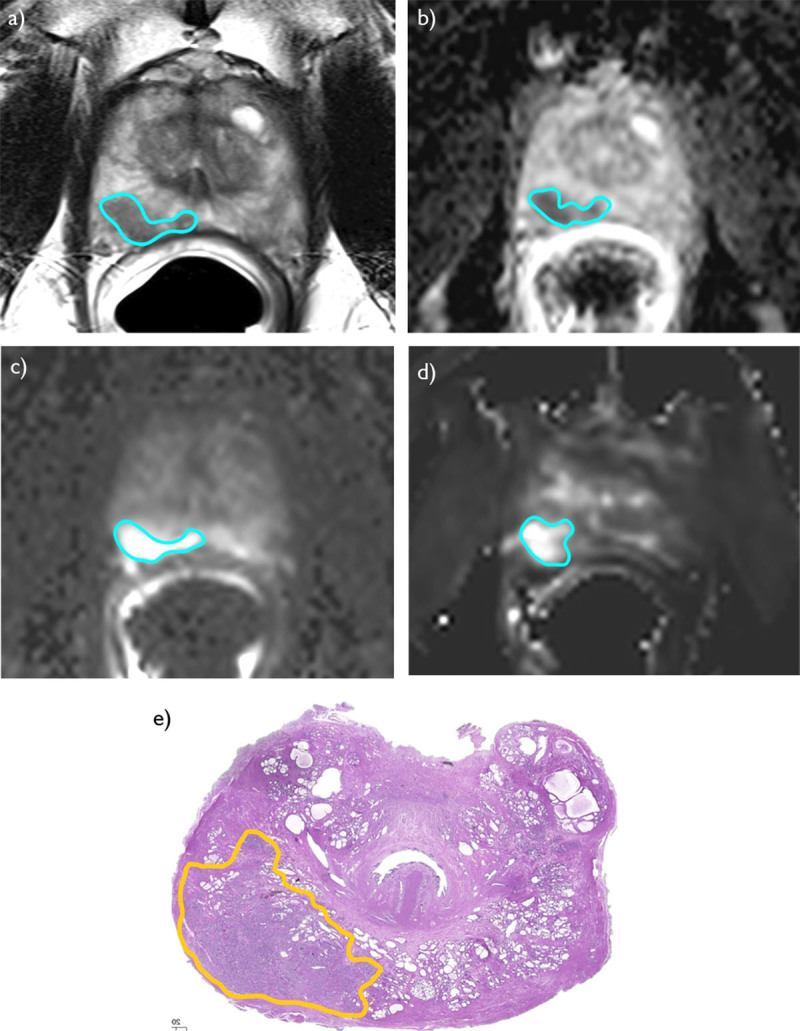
A 62-year-old man with Gleason Score 3 + 4 prostate cancer. The measurements of the index lesion volume on T2-weighted imaging (a), apparent diffusion coefficient map (b), diffusion-weighted imaging with a b-value of 2000 s/mm^2^
